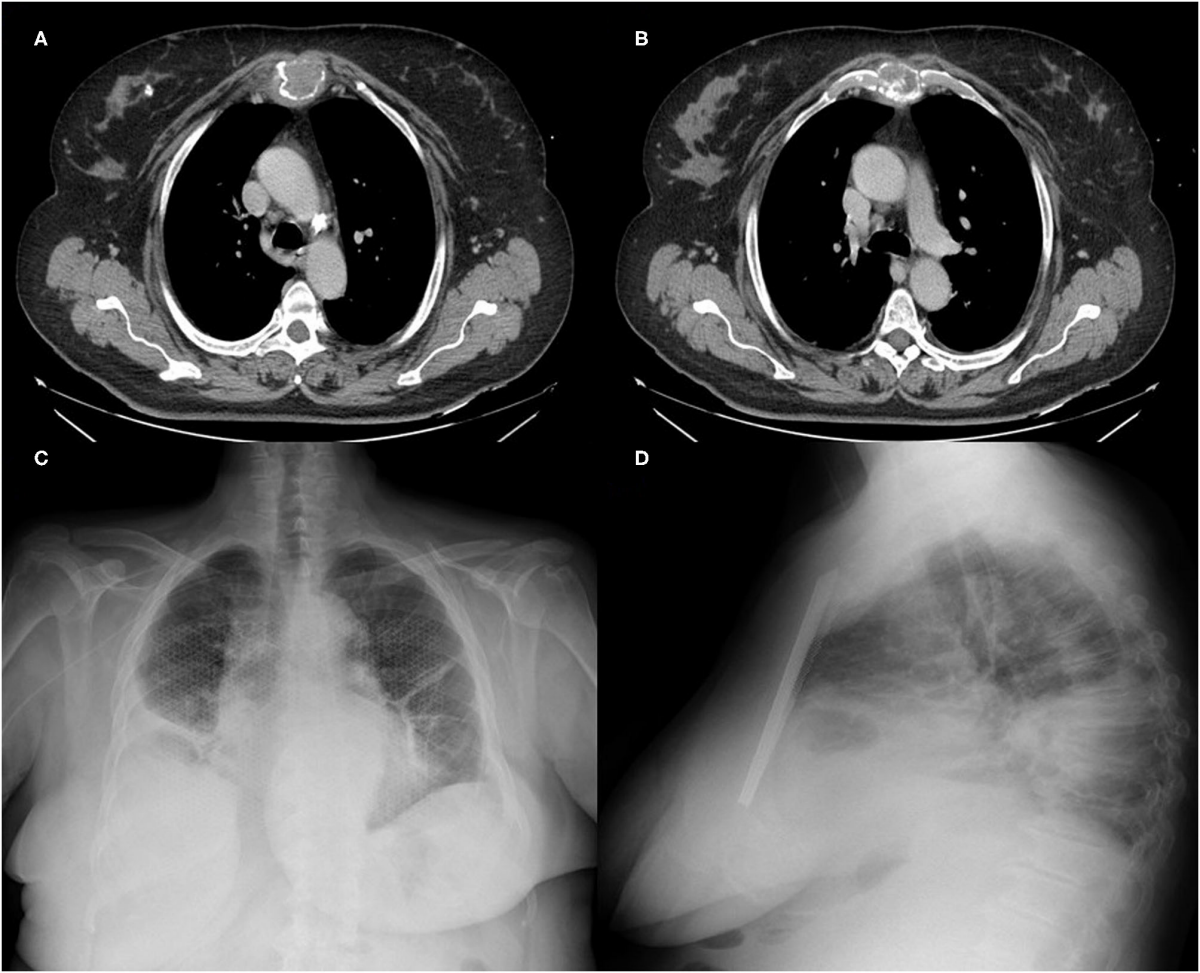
(A,B) Preoperative CT of the thorax showed a wide destruction of the upper 2/3 of the sternum by the neoplastic mass; (C,D) Chest X-ray at 40 days displayed the correct positioning of the titanium mesh, perfectly integrated into the wall.
